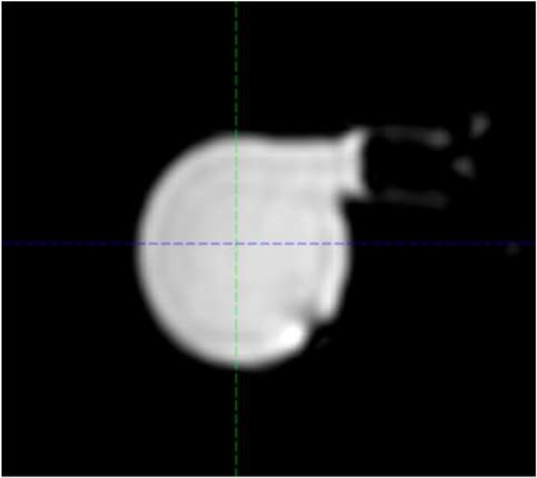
### Imaging with CT and MR
